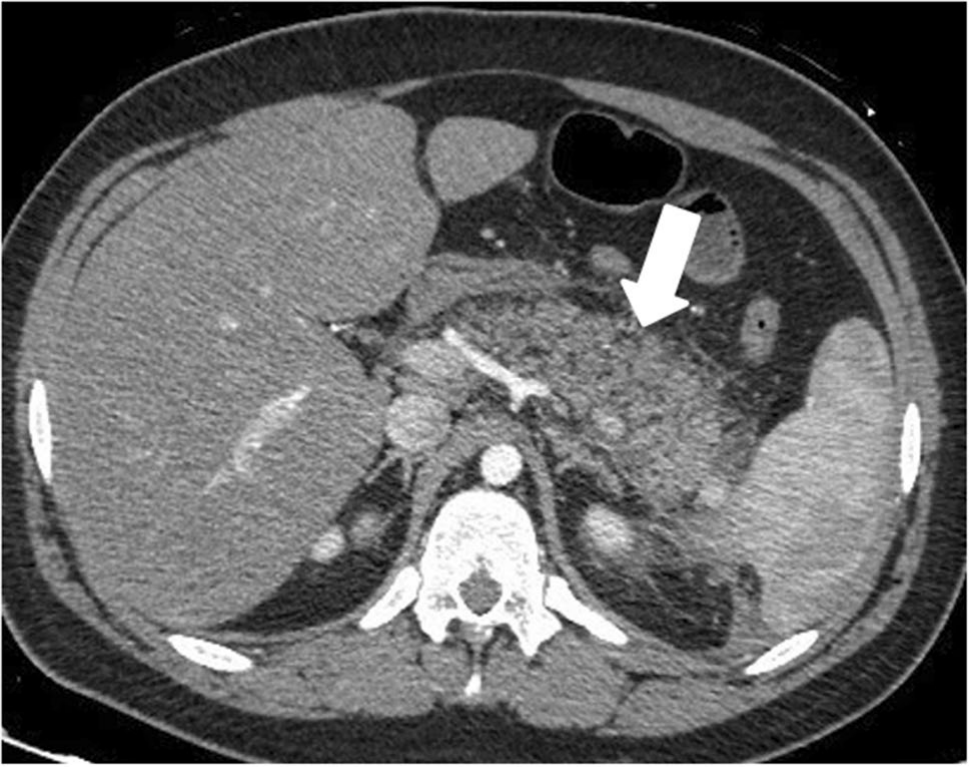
Interstistial acute pancreatitis in 17-year-old man, after cannabinoids abuse: axial IV contrast-enhanced CT scan shows mild diffuse enlargement of the whole pancreatic gland with poorly defined contours (arrow); the enhancement of the pancreatic parenchyma is normal and there are no foci of glandular necrosis
